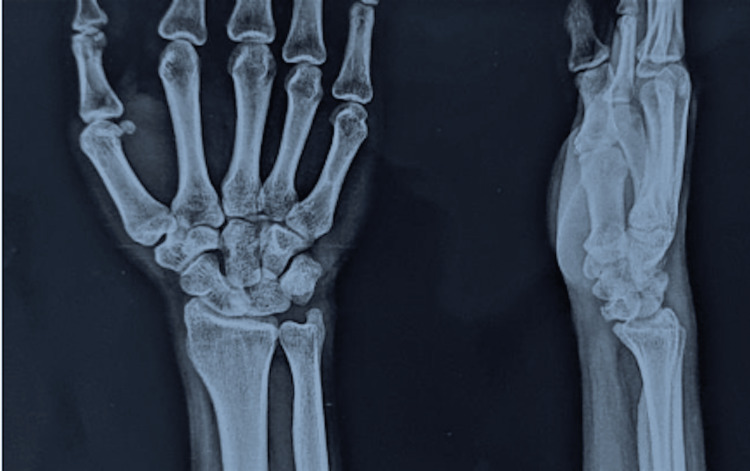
Preoperative X-ray showing a break in the proximal arc
